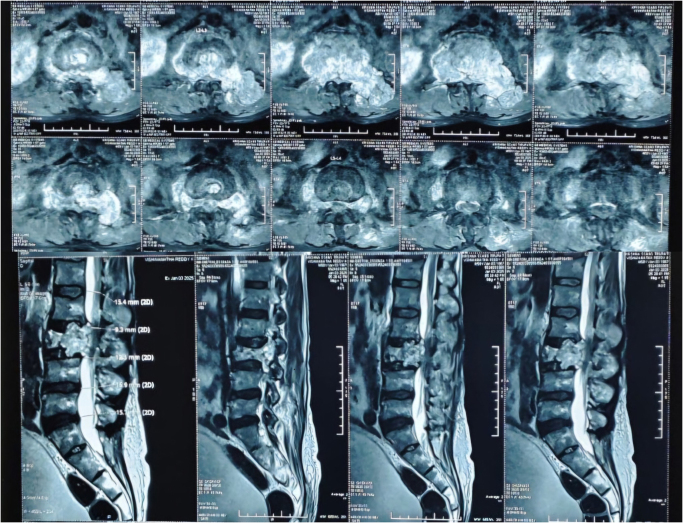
MRI scans showing axial (top) and sagittal (bottom) views of the lumbar spine. A bony mass is visible, likely indicating a lesion compressing the spinal canal. MRI, magnetic resonance imaging.
